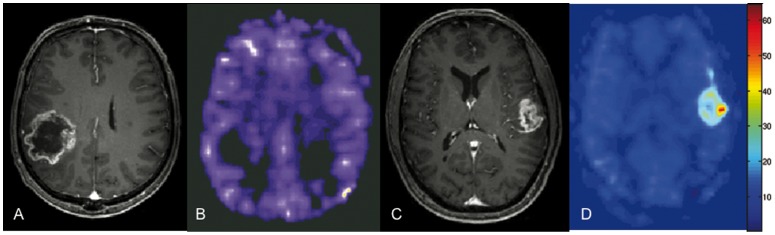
Contrast enhanced T1-weighted images (a, c) with corresponding ASL perfusion maps (b, d) in two glioblastoma patients. Figs. 4a and 4b show a low-perfused (mTBF = 97 ml/100 g/min) tumor, Figs. 4c and 4d depict a high-perfused (mTBF = 599 ml/100 g/min) glioma.
